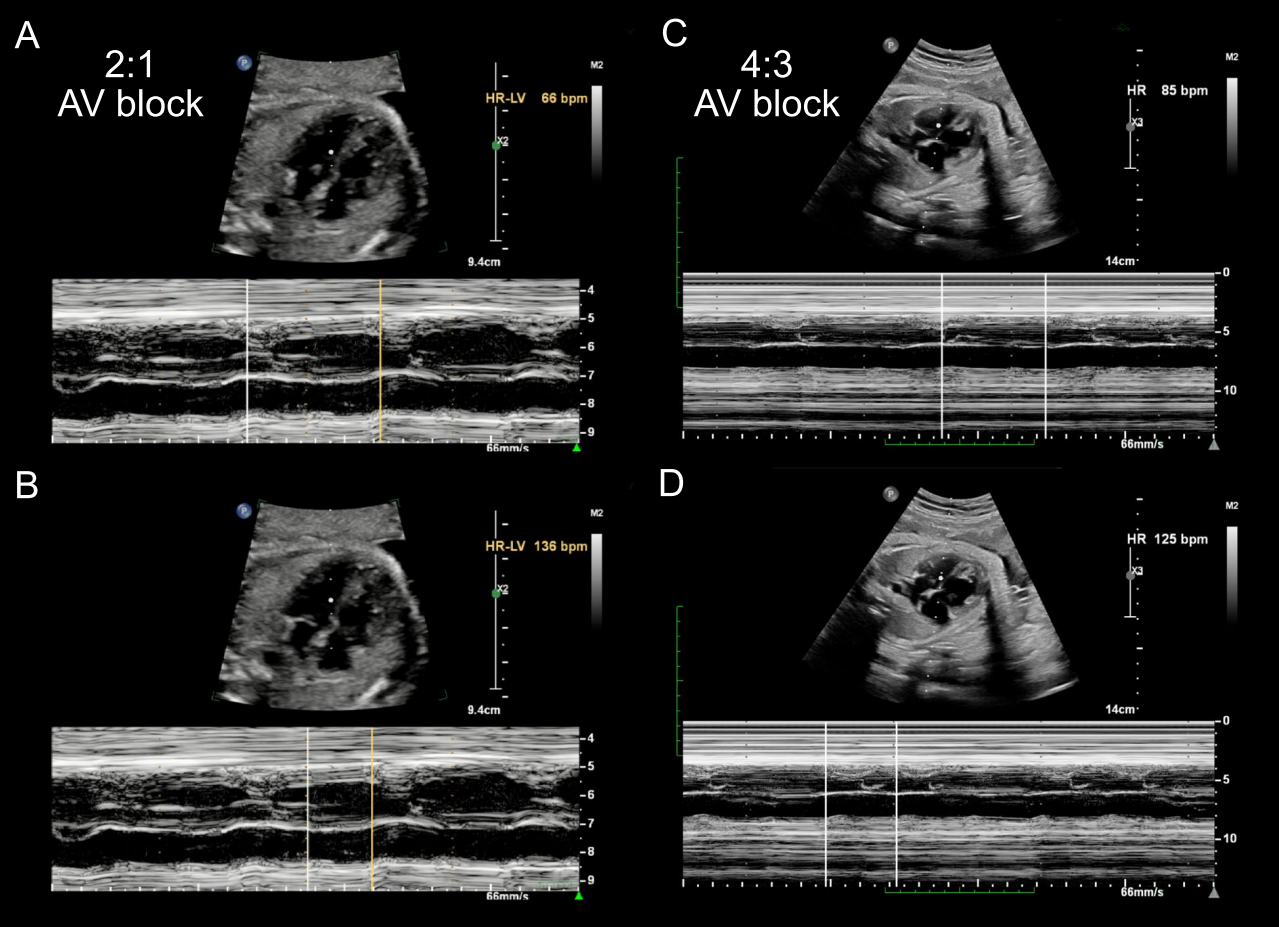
Fetal echocardiography to determine bradycardia. A M-mode echocardiography demonstrated the ventricular rate of 66 beats per minute before treatment. B M-mode echocardiography demonstrated the atrial rate of 136 beats per minute before treatment, indicating a 2:1 AV block. C M-mode echocardiography demonstrated the ventricular rate of 85 beats per minute after treatment. B M-mode echocardiography demonstrated the atrial rate of 125 beats per minute after treatment, indicating a 4:3 AV block
